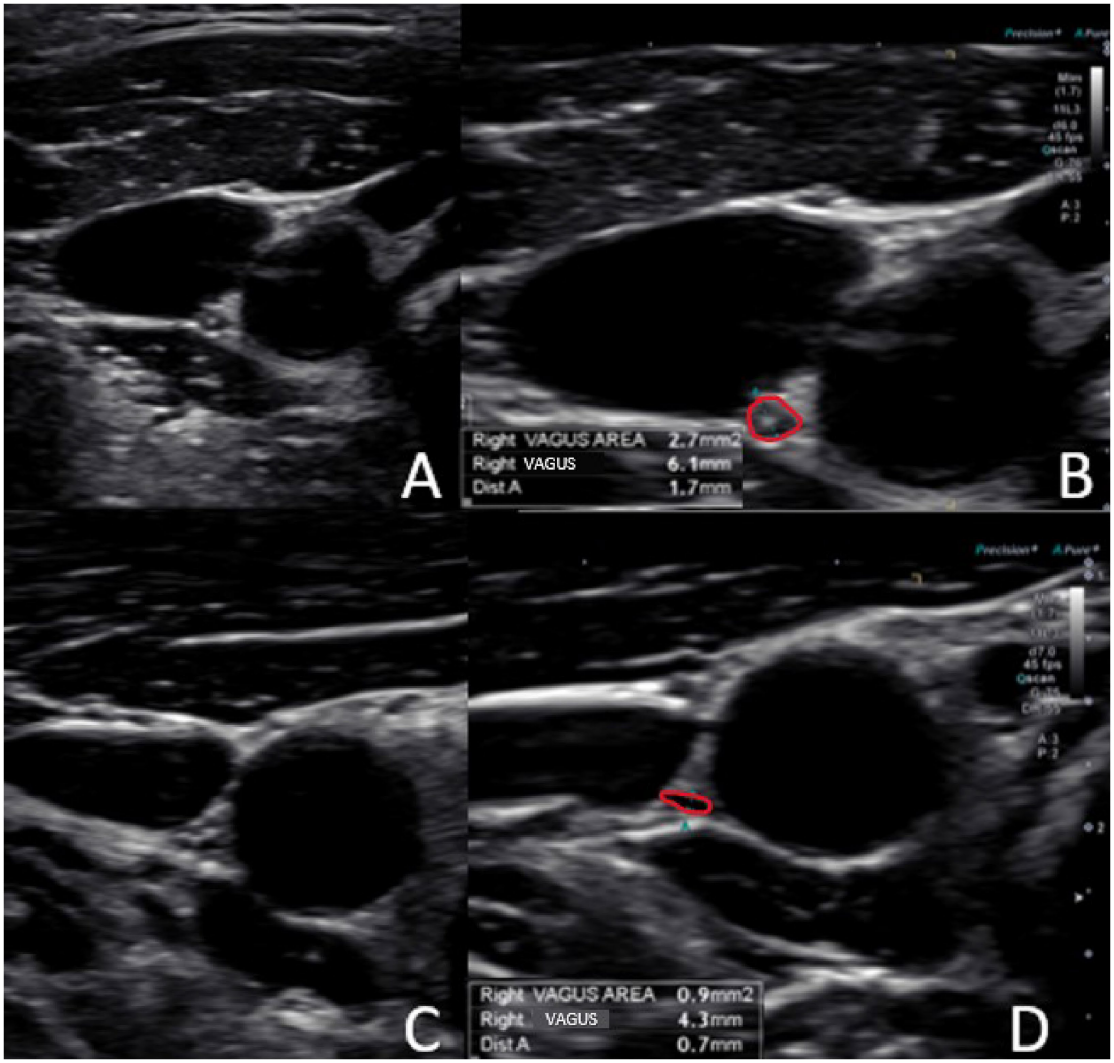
Ultrasound of the carotid sheath showing the vagus nerve. (A) Normal. (B) Normal image magnified and measured. (C) Degenerated vagus nerve. (D) Degenerated image magnified and measured.
